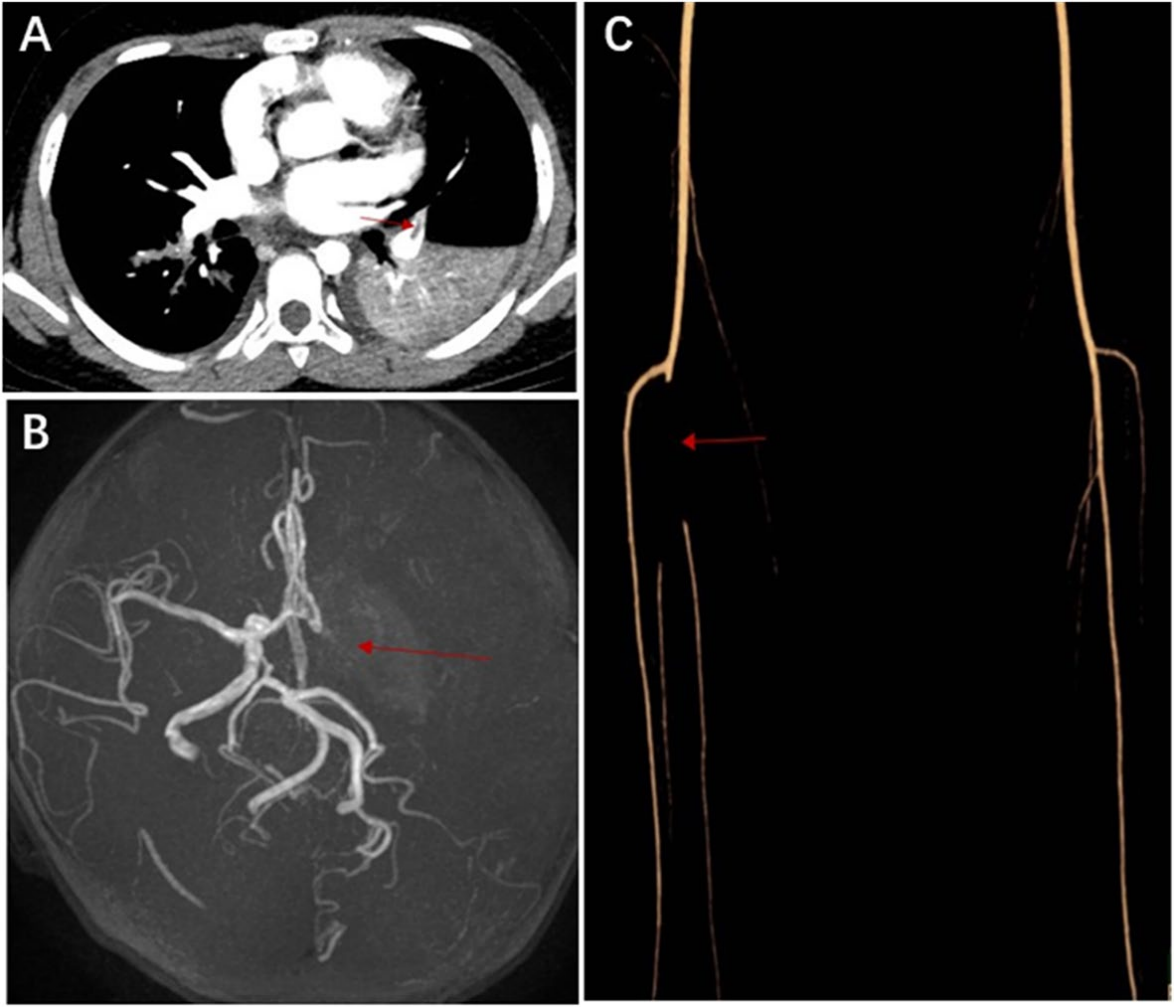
A Chest CTA showed a filling defect in the left upper pulmonary artery. B MRA examination of the head showed that the cavernous sinus segment of the left internal carotid artery, the middle cerebral artery and its branches were not developed. C CTA of lower limbs showed that the right posterior tibial artery was not developed locally
